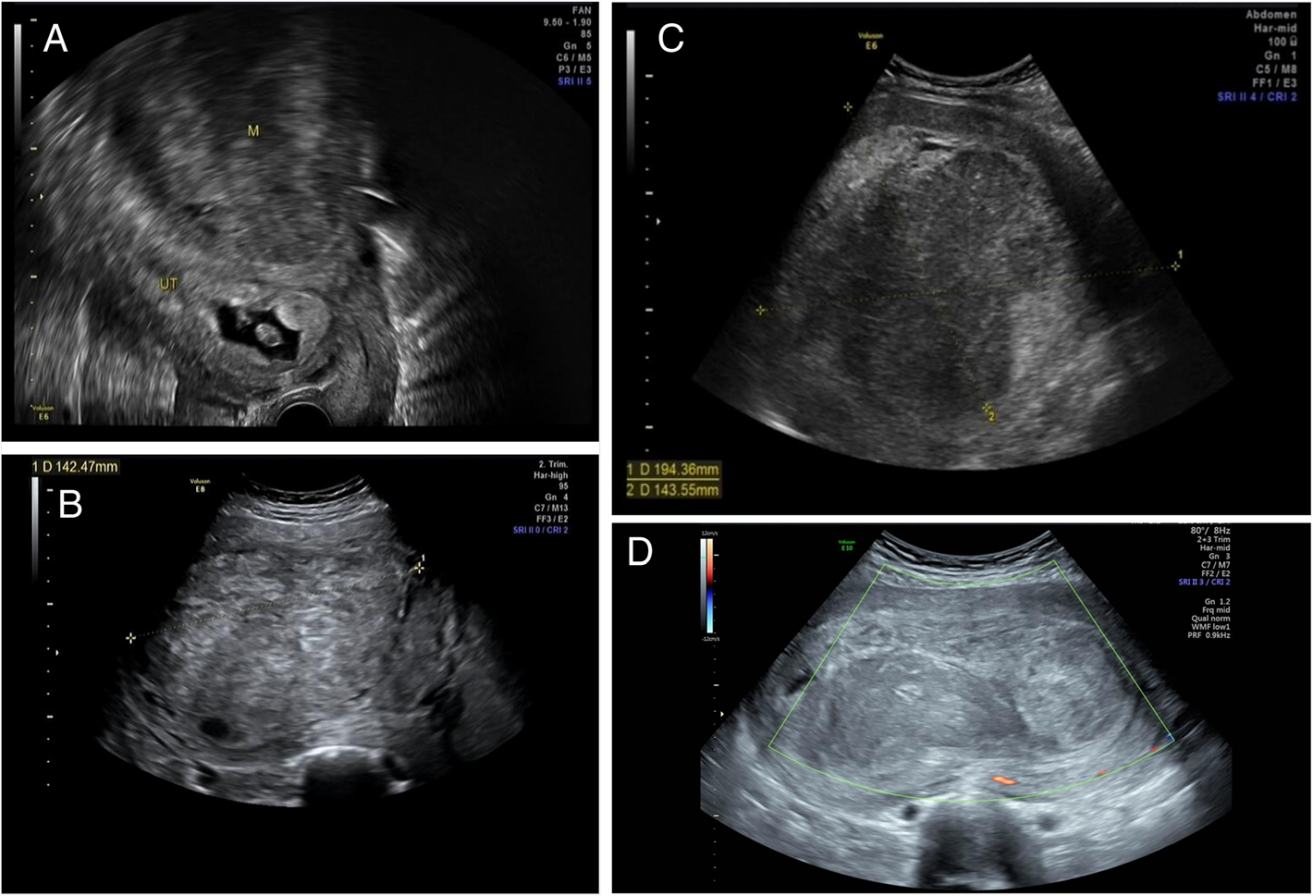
Ultrasound examinations of the fibroids during pregnancy. a Ultrasound showing an intrauterine pregnancy and an intramural posterior fundal fibroid; b Ultrasound of the original fibroid and two additional fundal fibroids; c Large posterior wall fibroid pushing the endometrium anteriorly; d Cross-section through the three submucosal myomas. The areas filled with fluid demonstrated a hyperechogenic pattern, which indicated the possibility of degeneration and necrosis
